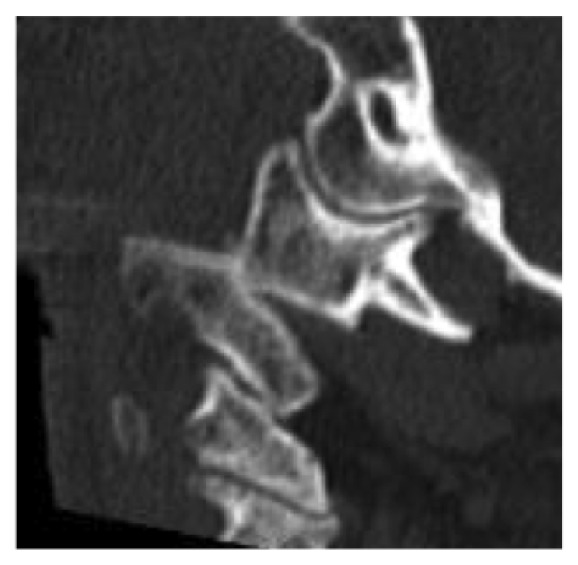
Sagittal CT reconstruction C0 to C3 demonstrating dorsal dislocation of C1 against C2.
